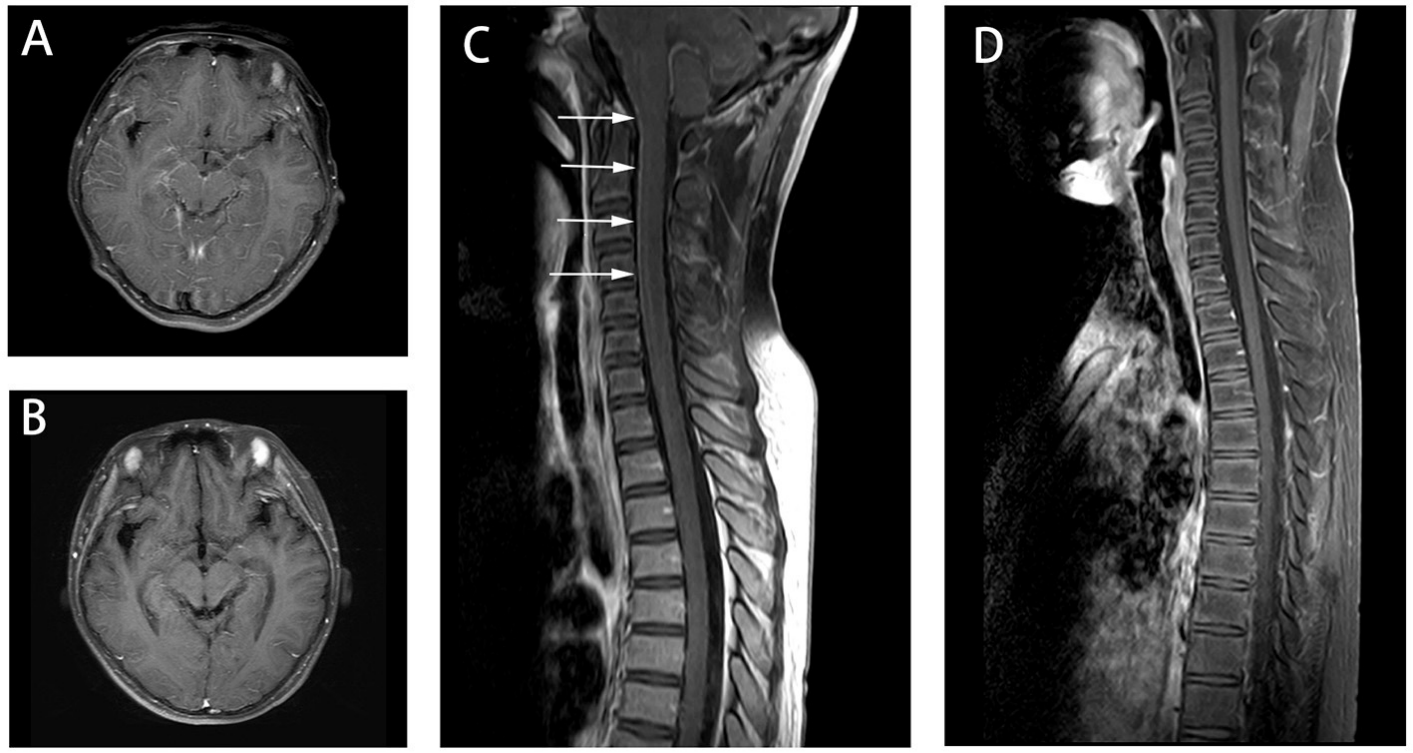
Axial fluid attenuation inversion recovery (FLAIR) MRI brain (A) 7 days after admission and (B) 3 weeks later. Image (A) showed the enhancement in the surface of the large and small brain as well as brainstem (not shown). Image (B) showed meningeal enhancement was reduced. Spine MRI (C) 10 days after admission and (D) 3 weeks later. Image (C) showed the enhancement of spine. And Image (D) showed the enhancement was reduced after the immunotherapy treatment.
